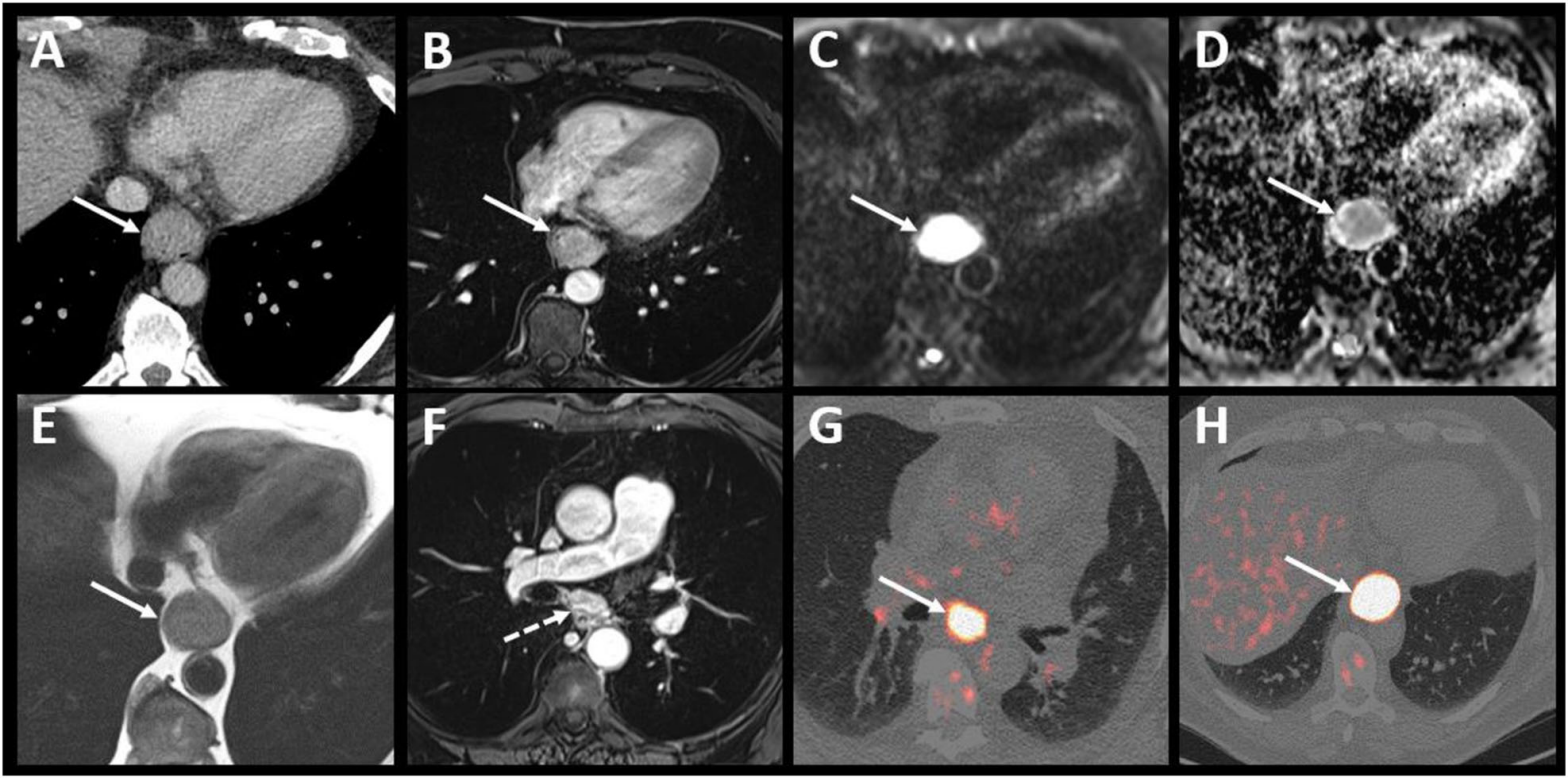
Example 3—High-quality soft tissue contrast in MR. A 60-year-old patient with adenocarcinoma located in the middle third of the esophagus, with CT image (A) suggesting a possible pleural invasion (white arrow). T1-enhanced MR (B) and diffusion-weighted (C) with ADC map (D) images clearly depict a circumferential esophageal tumor. The T2 MR image (E) shows a thin, fat interface between the tumor and the pleura, allowing pleural invasion to be ruled out, thus classifying the tumor as T3. T1-enhanced MR image (F) also depicted a small adenopathy in contact to the tumor (dotted arrow) classified the tumor as N1. This small lymph node was difficult to visualize on PET/CT images (G, H) due to the overlap of tracer fixation between the primary tumor and the adenopathy
